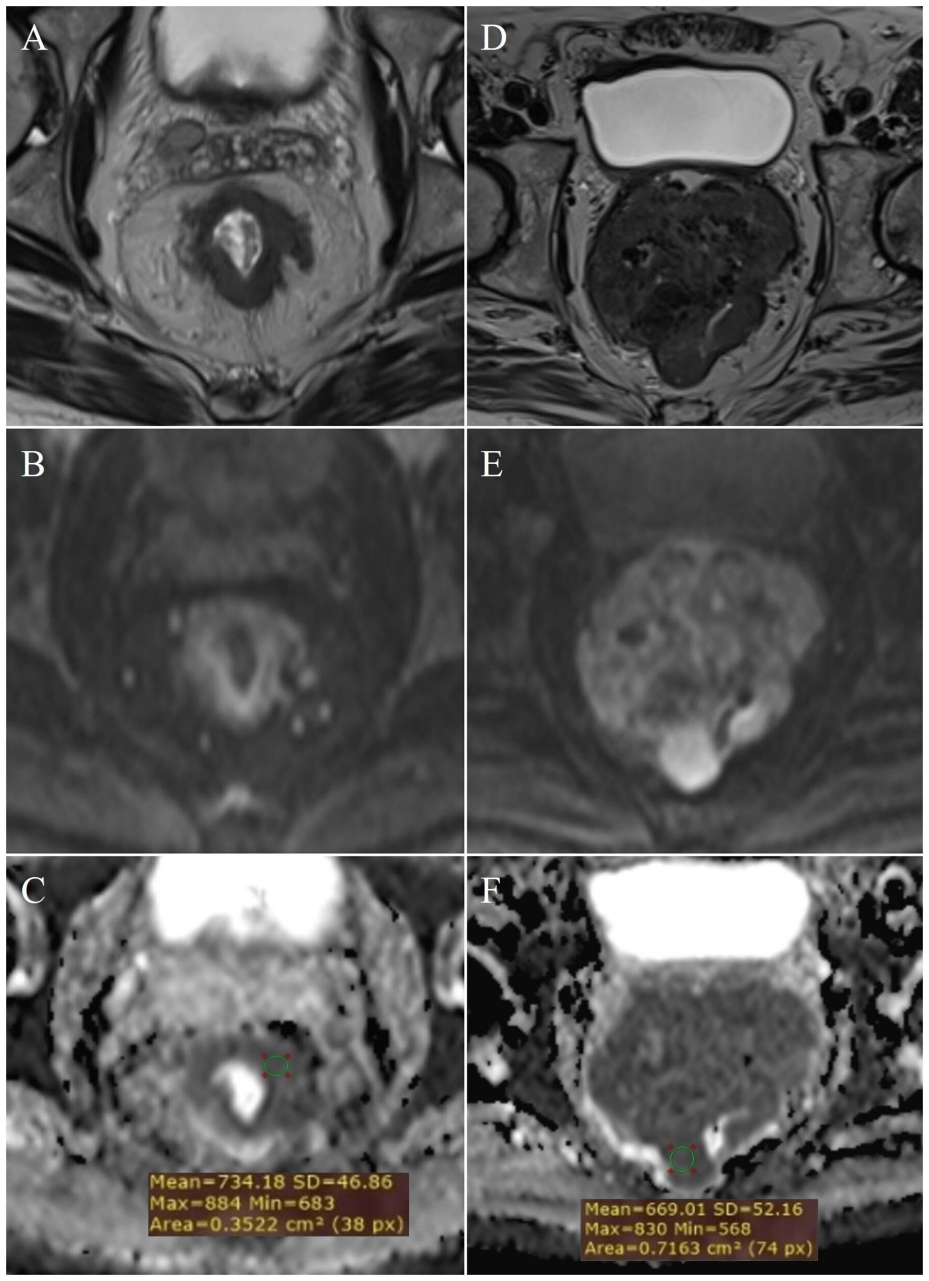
Measurement of ADC values: T3 stage of rectal cancer - (A) (T2W), (B) (DWI), (C) (ADC map with ROI); T4 stage - (D) (T2W), (E) (DWI), (F) (ADC map with ROI).
